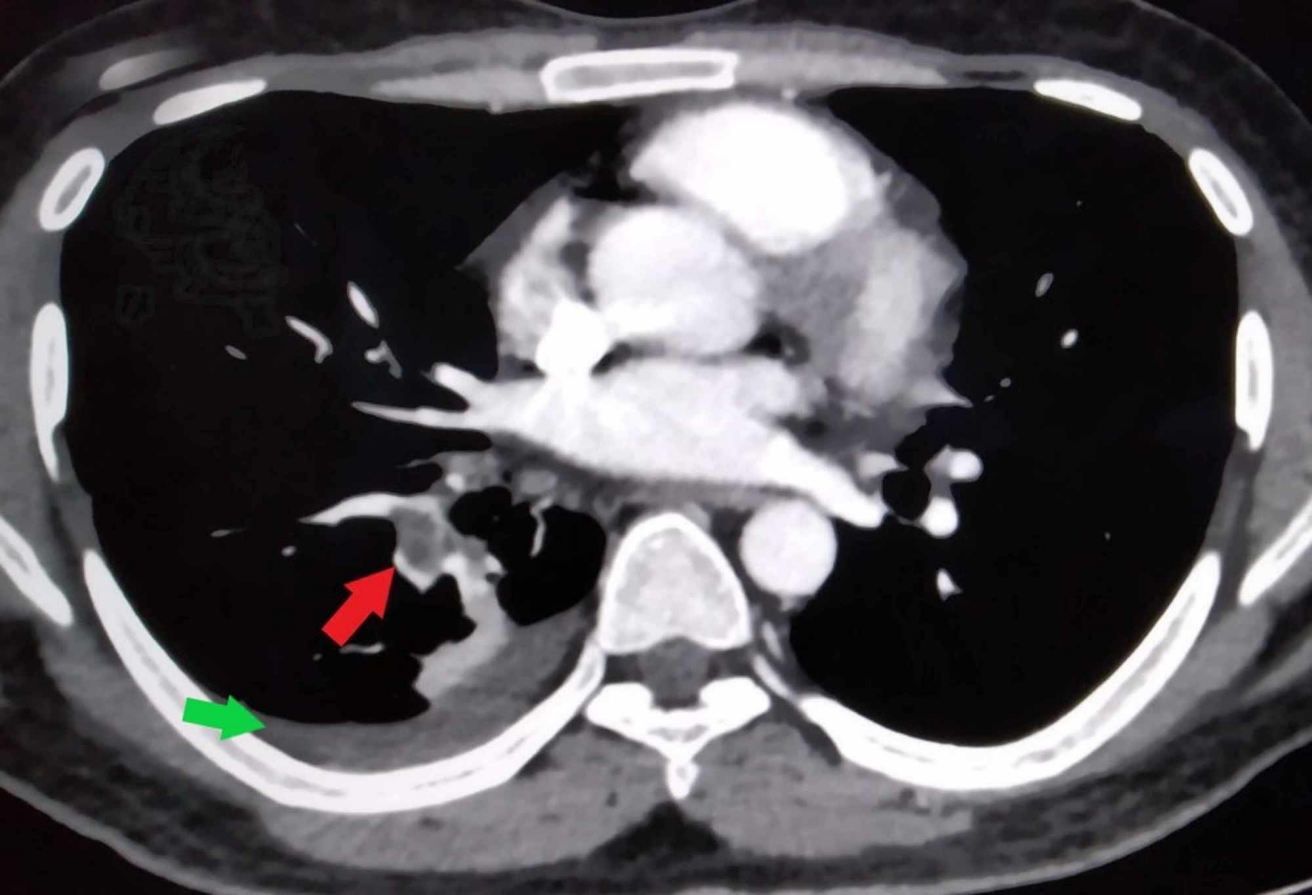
Axial section of computed tomographic (CT) angiogram of lungs showing pleural effusion (green arrow) and thrombus (red arrow) in a segmental branch of the right pulmonary artery.
